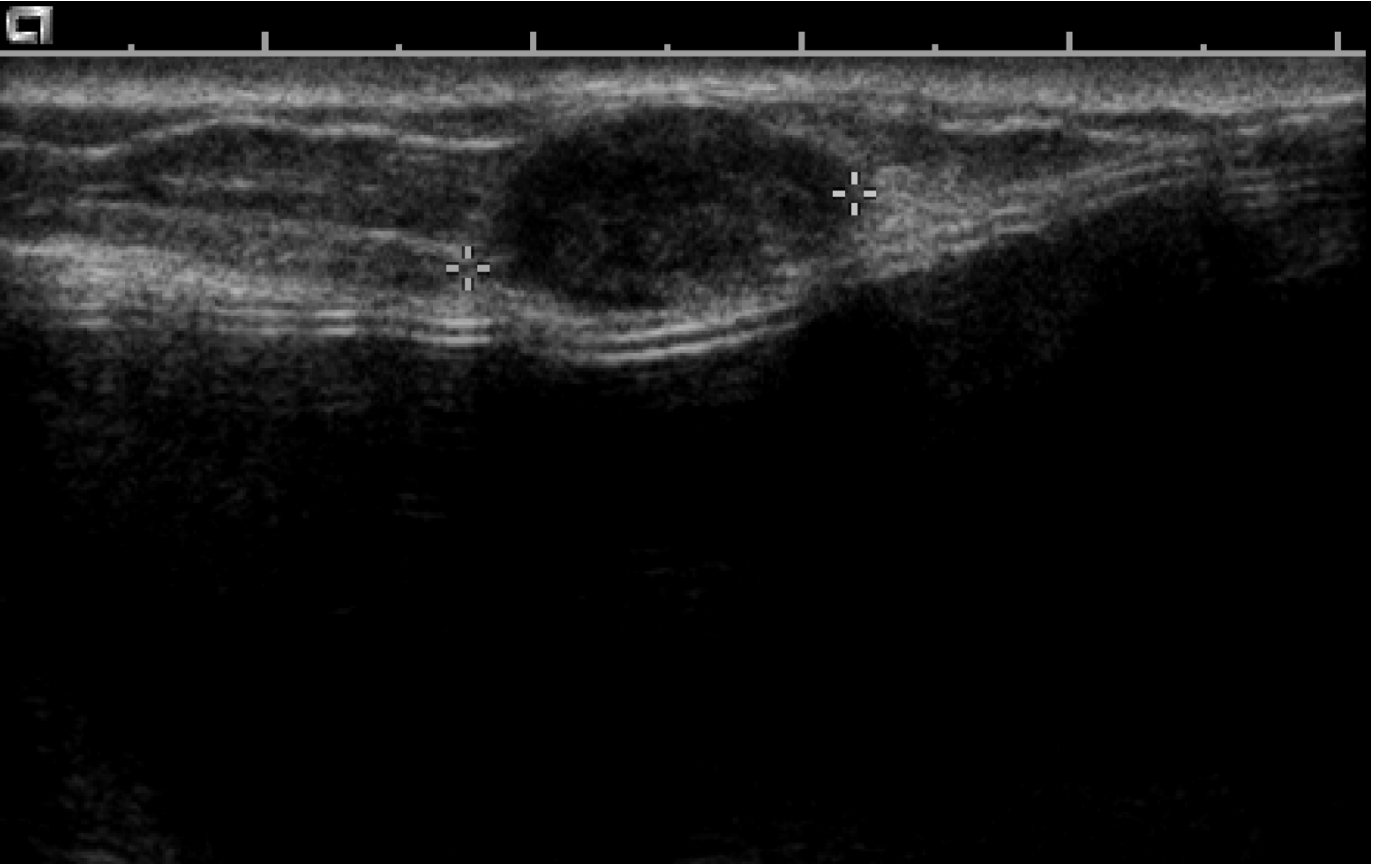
Sonographic examination: 15 mm hypoechoic solid, non-calcified circumscribed mass, with a thin echoic rim, benign in appearance, located in subcutaneous fat at 9 o'clock in the left breast.
